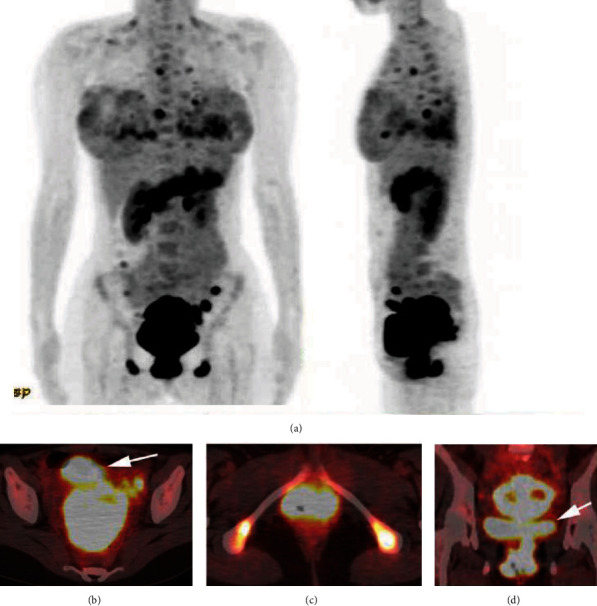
(a) FDG-PET/CT images of the whole body. Abnormal uptake of FDG was observed through the whole body, although it was strongest in the reproductive tract. (b) Axial FDG-PET/CT images of the uterine body. Urine (arrow) obscured FDG accumulation in the reproductive tract, whereas the uterus and the left ovary showed clear uptake of FDG. (c) Axial image of the ischial tuberosities and the vulva. (d) Coronal image of the uterus and the vagina. Excreted FDG into the bladder (arrow) was overlaid on the uptake in the reproductive tract.
